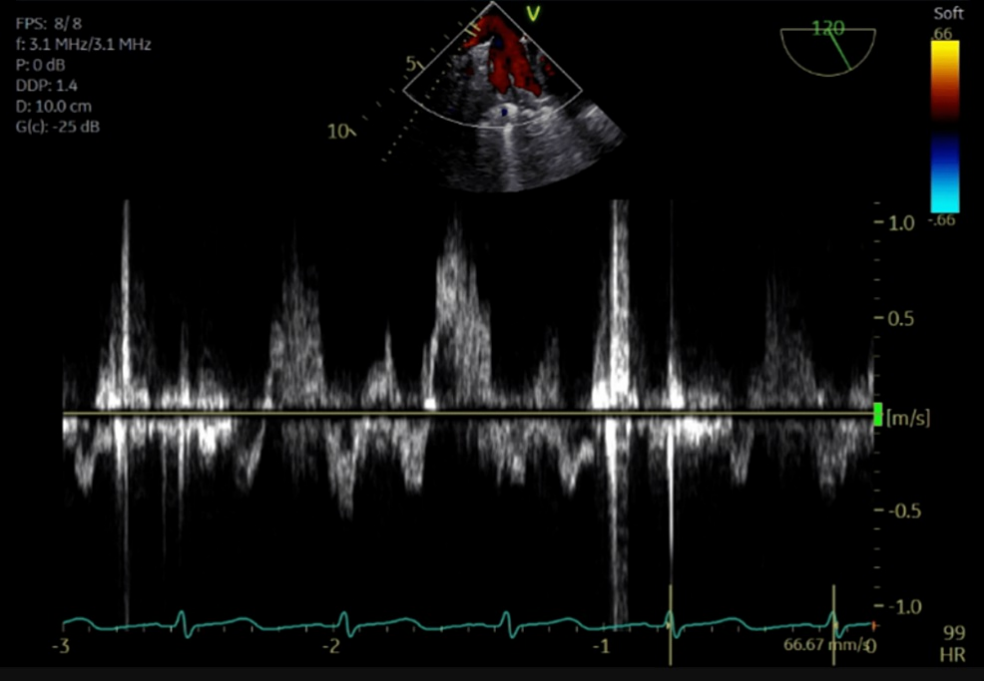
Pulsed-wave Doppler of the left inferior pulmonary vein showing systolic flow reversal.
